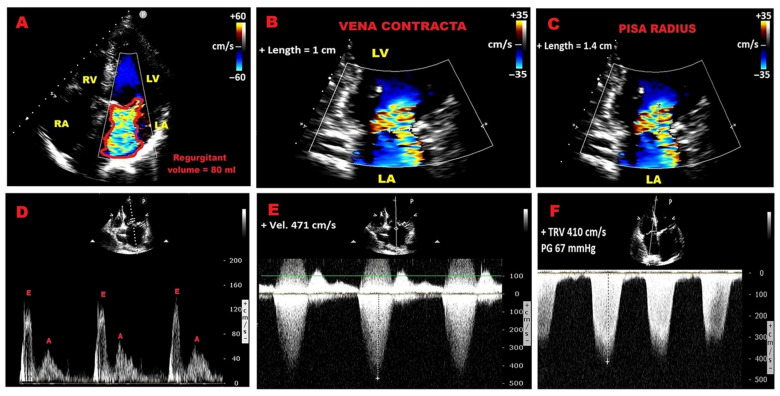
Echocardiographic predictors of adverse outcomes in primary mitral regurgitation, derived from color Doppler, PW–Doppler and CW–Doppler. (A) Regurgitant volume. (B) Vena contracta width. (C) PISA radius. (D) Dominant transmitral E wave. (E) Dense holosystolic continuous wave Doppler MR jet. (F) Increased tricuspid regurgitation velocity. CW, continuous wave; PW, pulsed wave; MR, mitral regurgitation; PISA, proximal isovelocity surface area.
